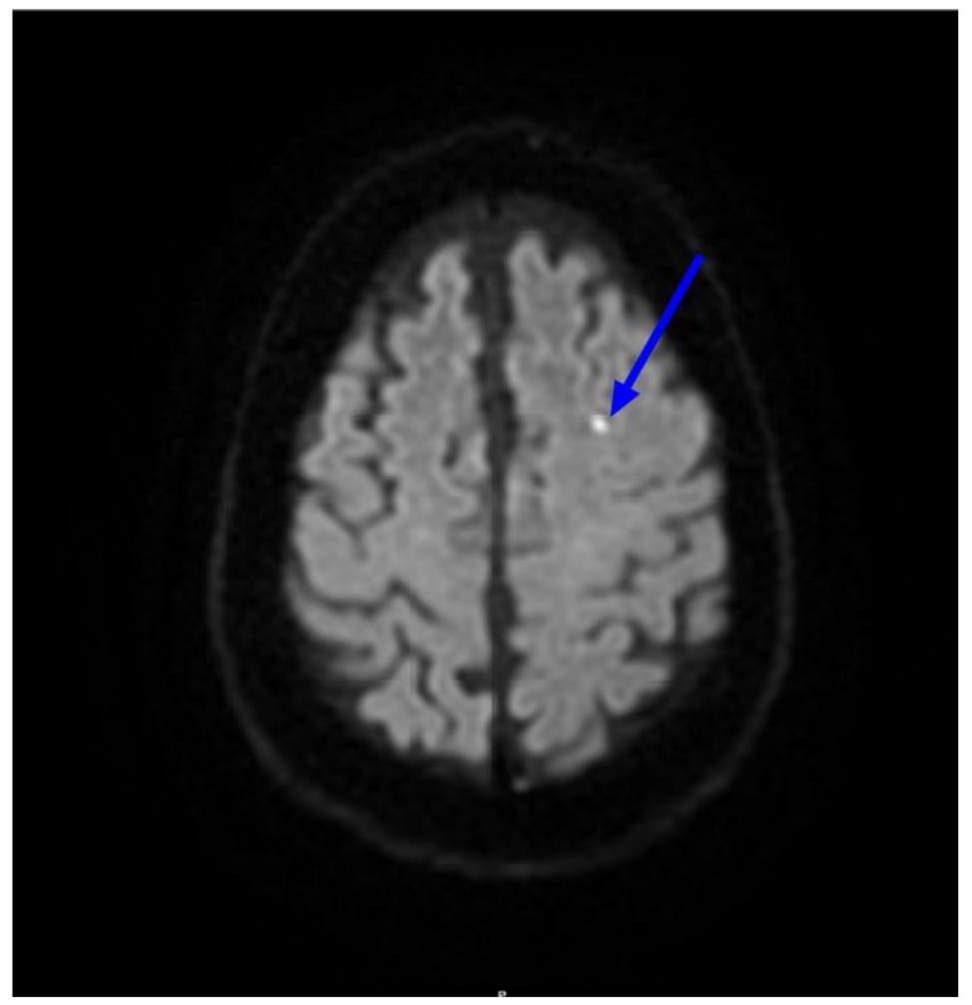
71 year old with a history of pernicious anemia. Presenting with fatigue and left sided temporal headache. Axial Diffusion weighted (DWI) MRI imaging of the brain demonstrates small focus of restricted diffusion in left frontal lobe consistent with acute or subacute infarct
